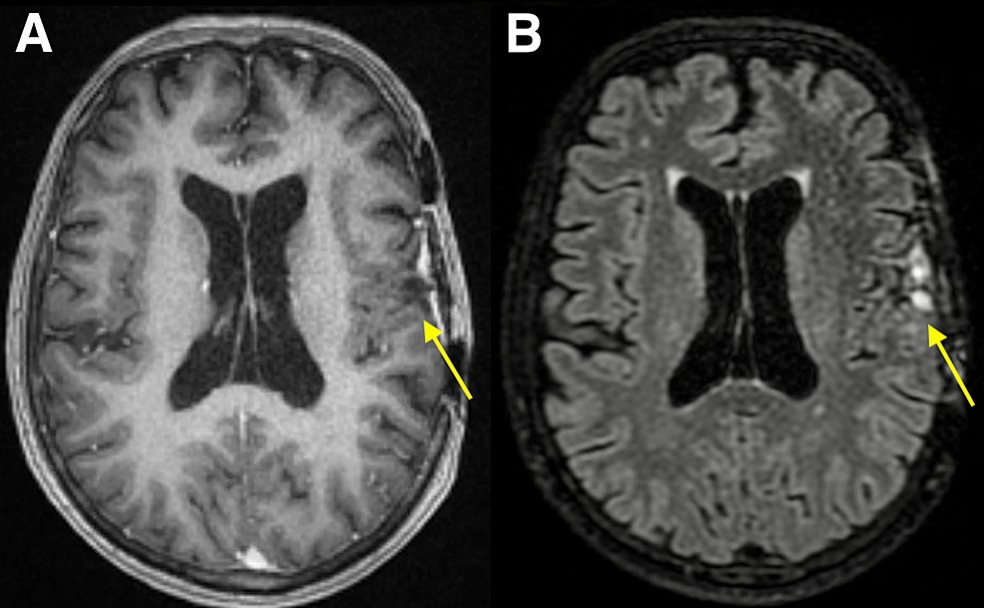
MRI 93 months after Gamma Knife stereotactic radiosurgery, 6 months after second resection. Axial contrast-enhanced T1-weighted (A) and T2-weighted FLAIR brain MRI (B) obtained six months after the second resection of cavernous malformation, demonstrating postsurgical changes from left frontal cavernous malformation resection with the resolution of left cerebral hemisphere vasogenic edema and midline shift. No evidence of residual or recurrent lesion or intracranial metastases. FLAIR: Fluid-attenuated inversion recovery
